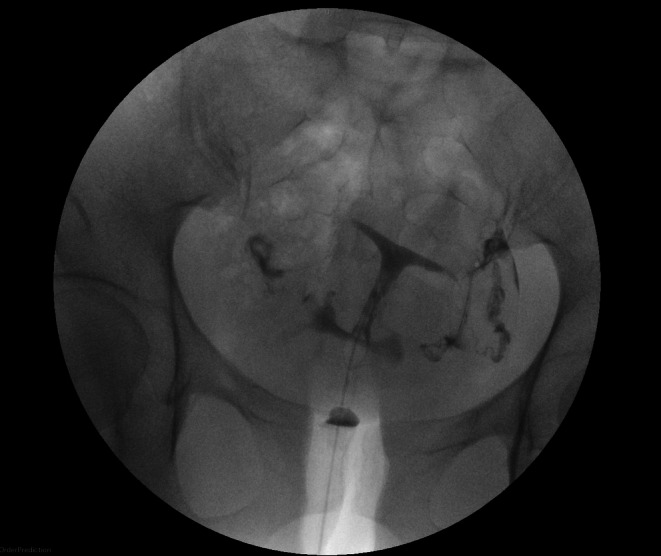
Hysterosalpingography (HSG) of a T‐shaped uterus. Fluoroscopic image acquired during contrast medium injection revealing a tubular endometrial cavity with minimal lateral expansion and a flat or indented fundal contour, consistent with a T‐shaped configuration. Bilateral tubal patency is also observed. This radiographic finding supports the diagnosis of dysmorphic uterus and guides subsequent surgical planning.
